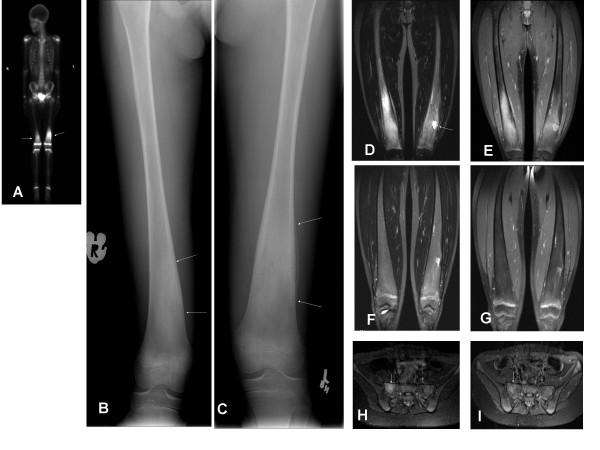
Imaging data of CRMO involving both distal femurs in patient 2, an 11-year-old girl. (A-E) Pre-treatment imaging: (A) Pre-treatment technetium^99^nuclear bone scan demonstrates abnormal uptake in both distal femurs with more prominent changes on the left (arrow). (B-C) Anterior-posterior radiographs of both distal femurs demonstrate ill-defined areas of sclerosis (arrows) mixed with small focal lucent areas. Periosteal reaction is noted on the left side. (D) Coronal STIR MRI images obtained 4 months after the bone scan reveal increased signal in the right femur. The left femur reveals post-operational changes on the site of previous bone biopsy (arrow). (E) Post-gadolinium fat-saturated T1 weighted image shows marked enhancement of the right distal femoral lesion, with post-operational changes in the left femur. (F-G) Imaging 10 months after initiation of pamidronate: Complete resolution of the right femoral lesion is demonstrated on STIR image (F) and post-gadolinium fat-saturated T1 weighted image (G). (H) Imaging at clinical relapse: Coronal STIR MRI images reveal increased T2 signal in the right aspect of the sacrum, consistent with inflammation. (I): Imaging 2 months post 1 day pamidronate retreatment: Previously demonstrated abnormal signal on coronal STIR has resolved (arrow).
